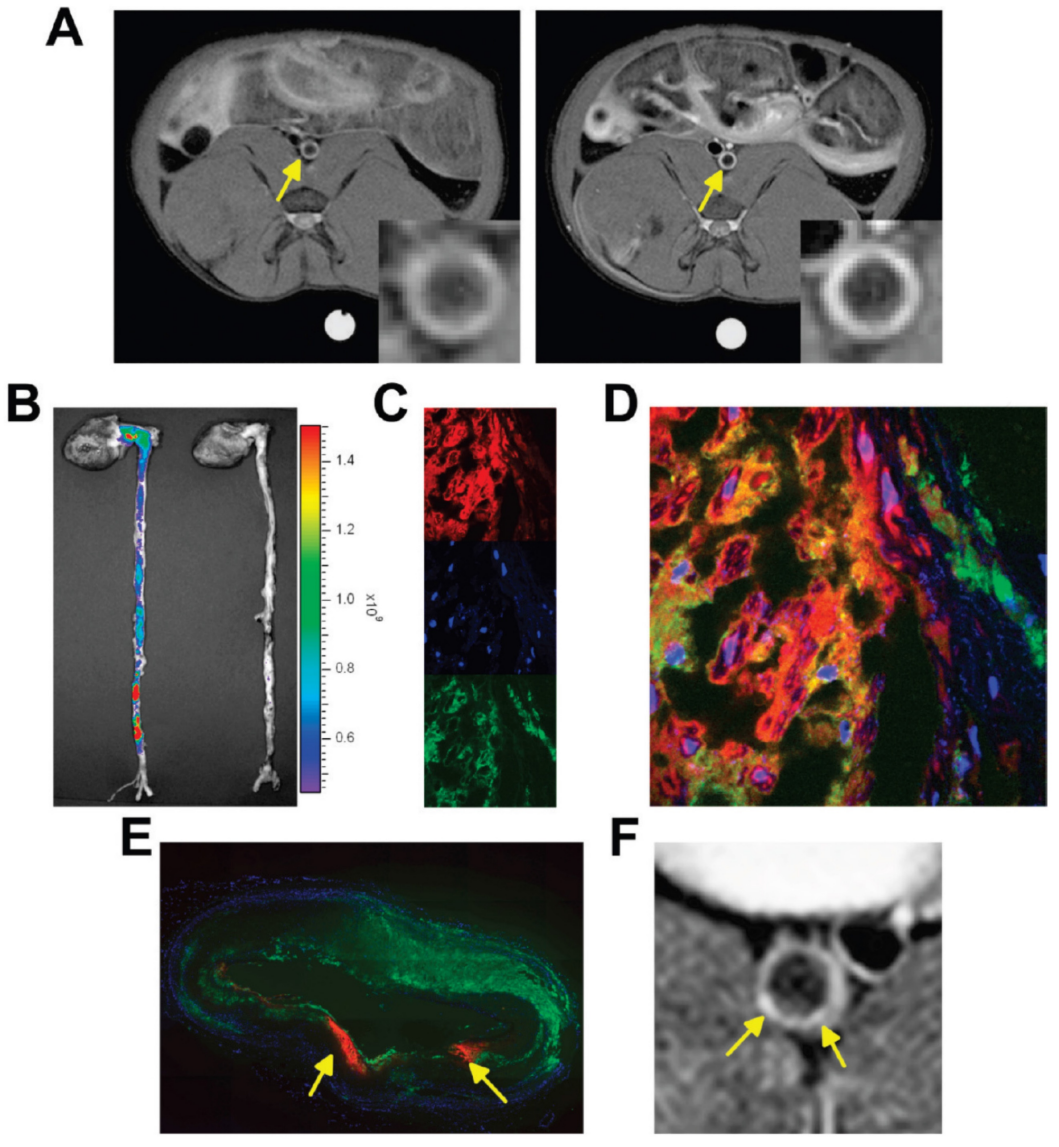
By using CLSM and MRI, images of the delivery and localization of liposomal PLP were obtained. Reproduced with permission from ref. 112 Fig. 2, (ACS Publications, 2010©).
